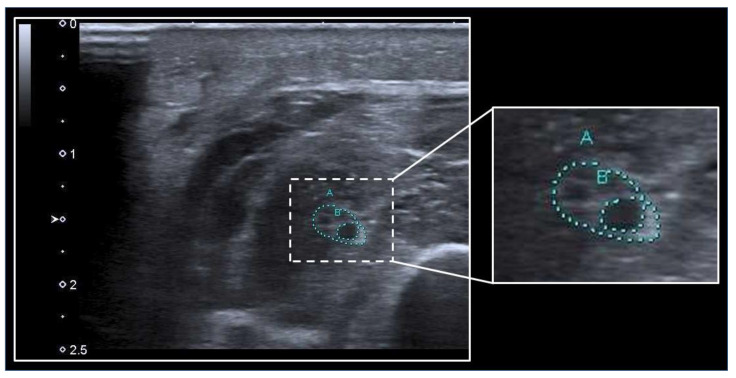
Assessment of nerve CSA with ultrasound. The median nerve (A) at the mid to proximal forearm is surrounded by muscles and can be identified by the heterogeneous “honeycomb” pattern within the echorich perineurium. A swollen fascicle (B) within the nerve depicts as a hypoechogenic area. The nerve CSA can be calculated using the tracing function of the ultrasound machine.
